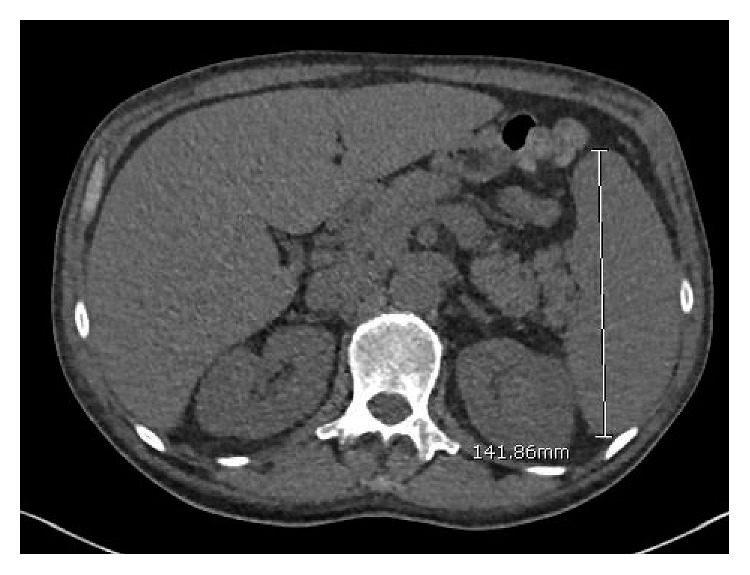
CT abdomen showing splenic enlargement of 14.8 cm.
